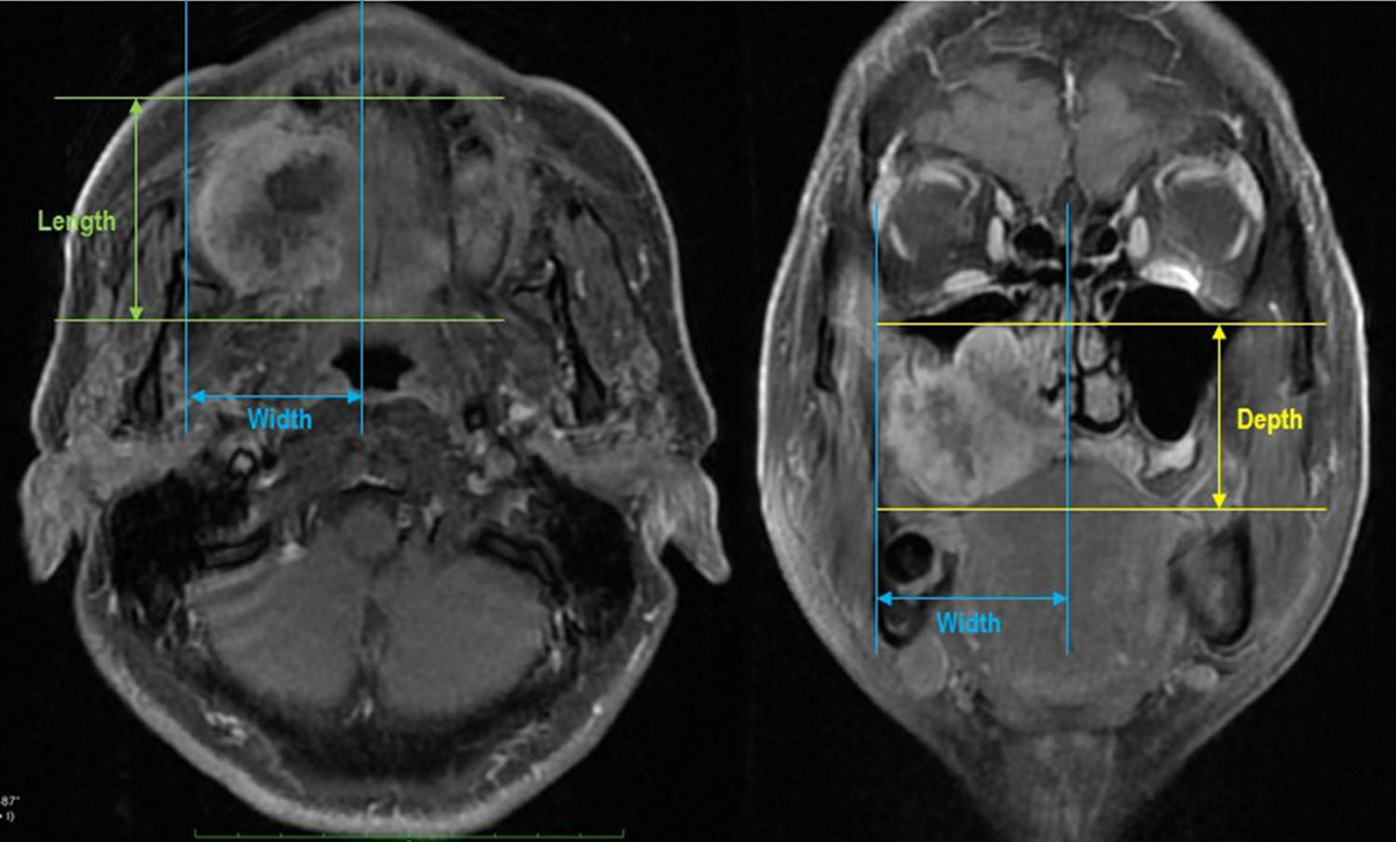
Orthogonal measurement of tumor size—metastatic patient. Tumor length and width were measured on axial MRI (left). Tumor depth and width were measured on coronal MRI (right). Both images were taken in a same patient where LN metastasis WAS found
